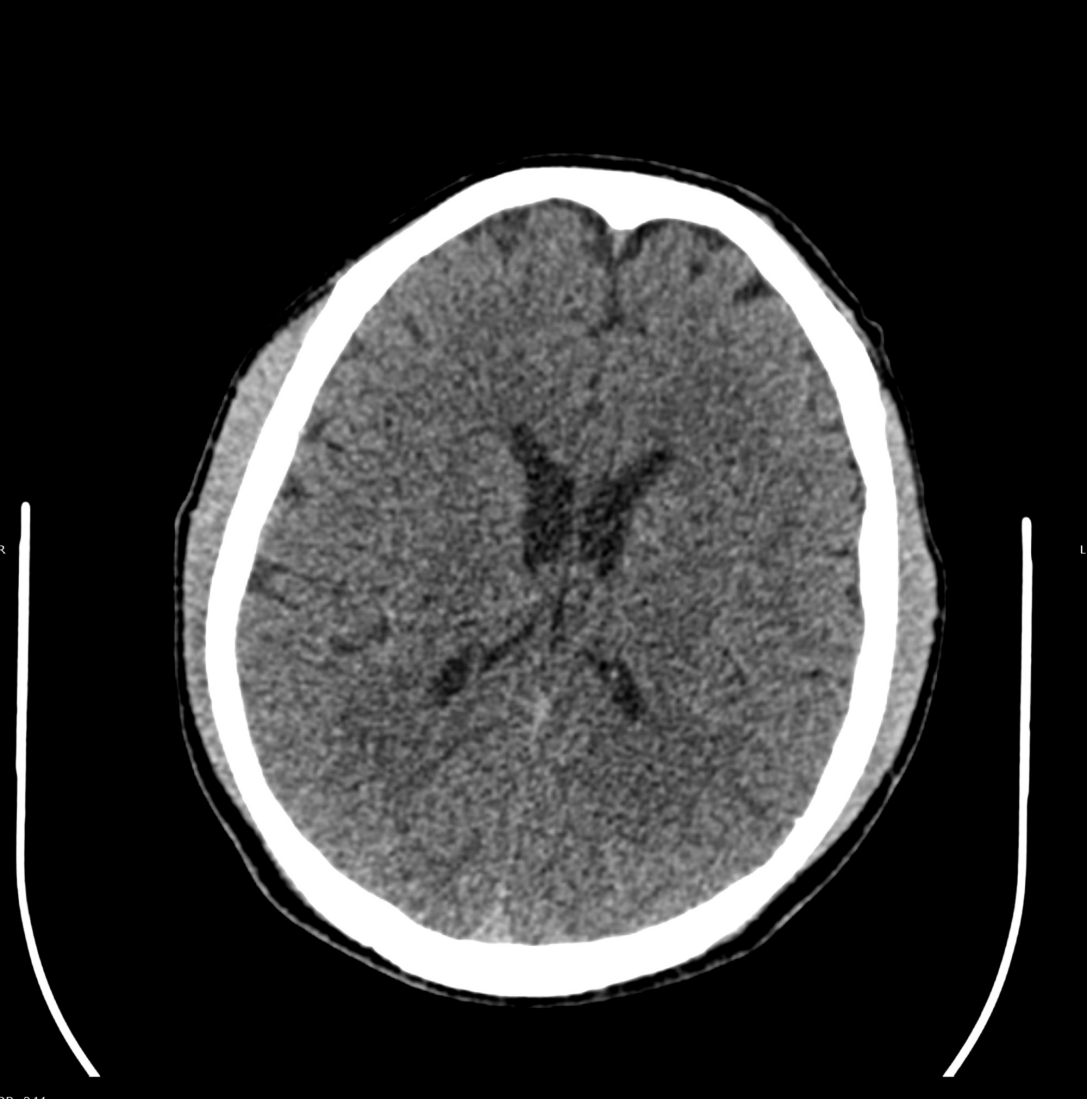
CT scan brain reported normal
